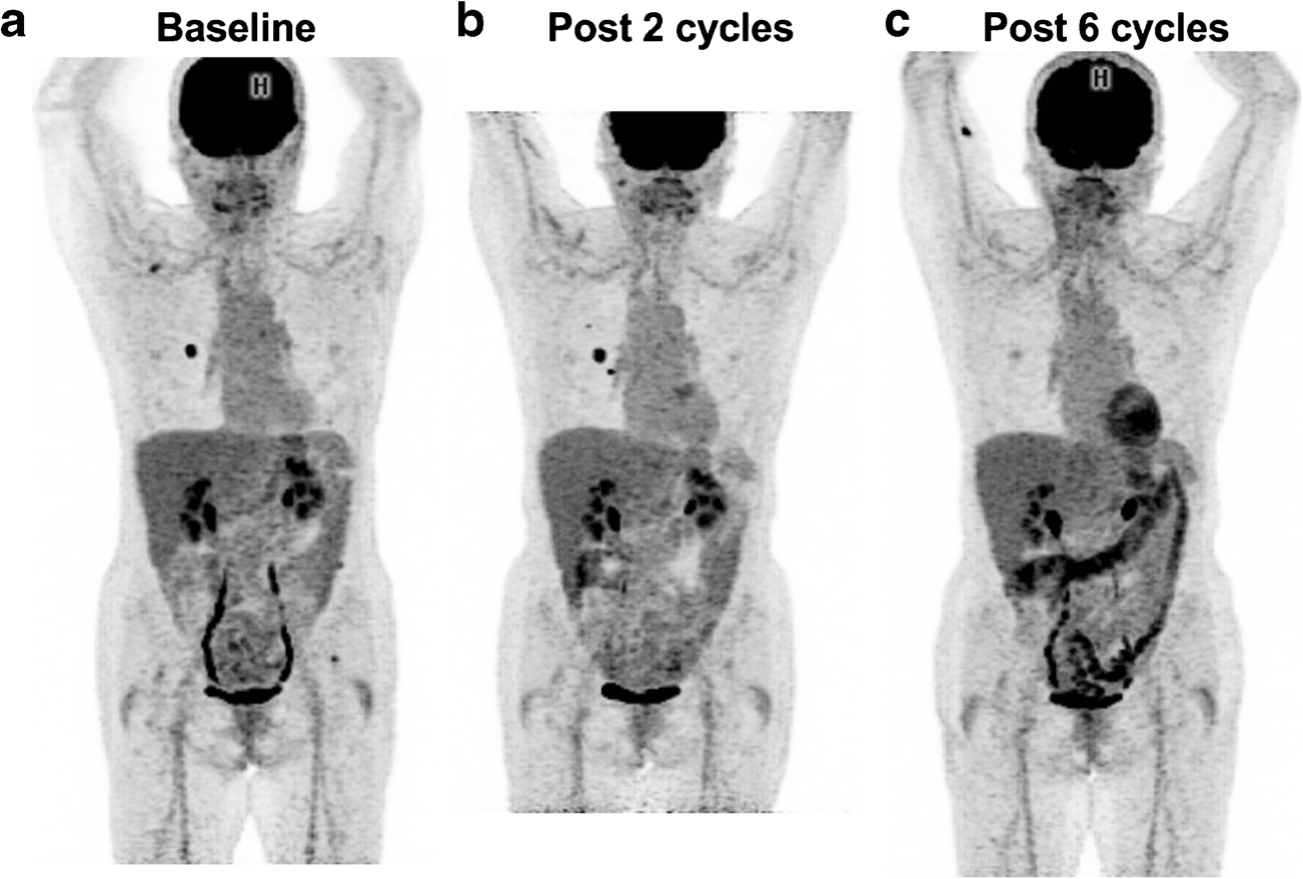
FDG PET images in a melanoma patient with lung metastases treated with nivolumab. a Baseline scan. b Early scan after two cycles shows two new lung lesions. c Scan after six cycles shows a complete metabolic response. Note the appearance of diffuse colonic uptake reported as possible colitis. The patient had no digestive symptoms. The progression seen after two cycles was considered to represent pseudoprogression
